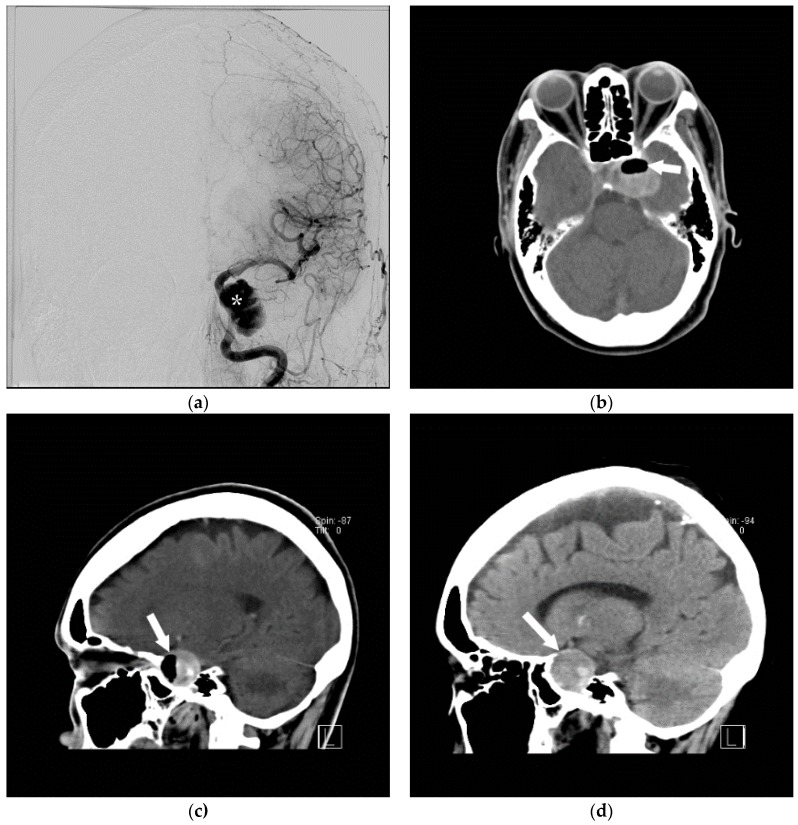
A 68-year-old woman underwent cerebral angiogram (a) for evaluation of 3 cm left cavernous internal carotid artery aneurysm (*). Post procedure, she developed transient aphasia, right facial droop, and right arm brachyplegia likely secondary to air embolism. This was presumed to be related to faulty occlusion balloon. The patient was immediately placed in a cervical collar to minimize head movements and distal air embolization as well as started on 100% oxygen therapy. Hyperbaric chamber therapy was discussed but declined due to concerns about patient movement during transport. CT angiogram (b,c) was performed immediately. Post-procedure revealed air within the large left cavernous segment internal carotid artery aneurysm (arrow). A CT performed 2 days later (d) showed the embolism had resolved and this patient made a full recovery.
